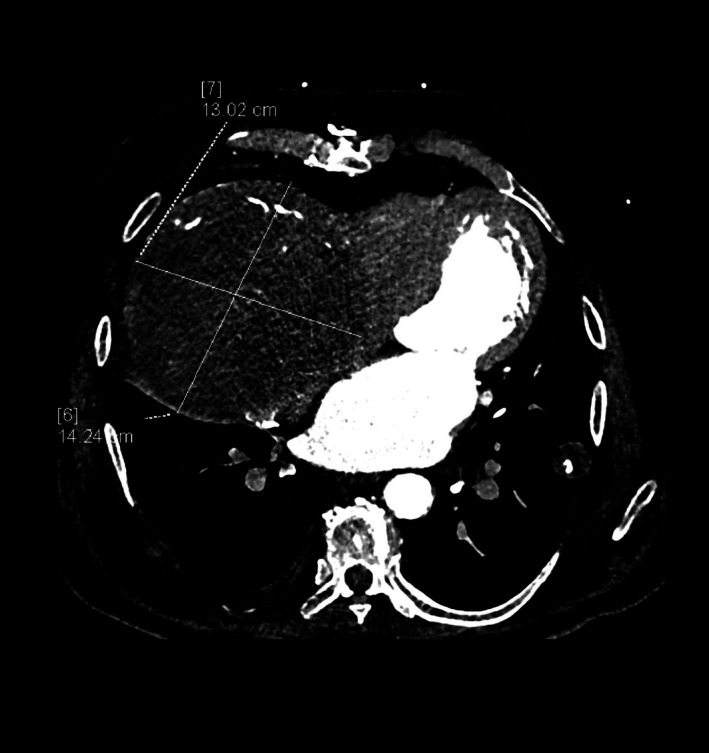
Coronary CTA, confirming giant coronary artery aneurysm (GCAA) of RCA.
